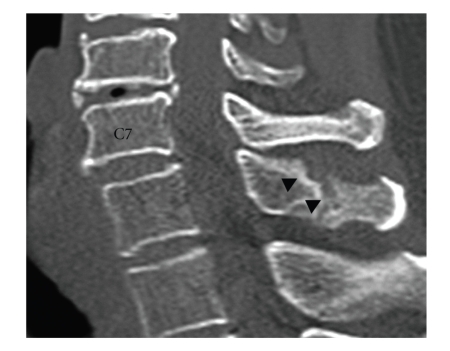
A sagittal reconstructed computed tomogram (CT). Nonunion of the T1 spinous process fracture (arrowheads) and slight anterolisthesis of C7/T1 are detected.
